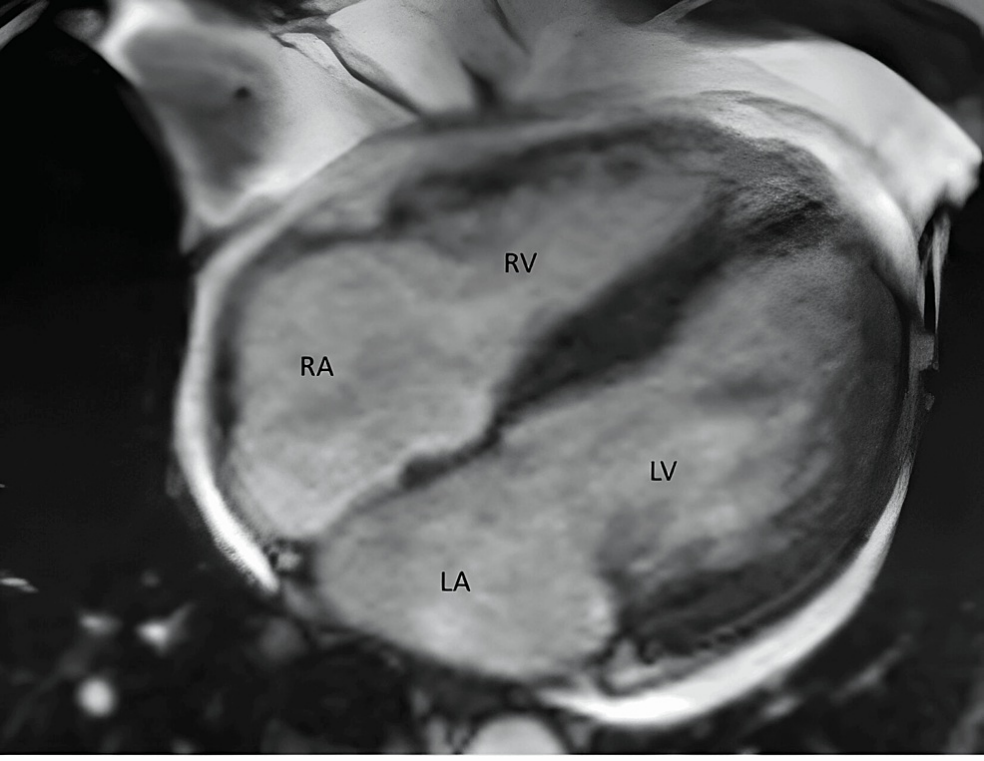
Cardiac magnetic resonance imaging, demonstrating normal systolic function RA, right atrium; LA, left atrium; LV, left ventricle; RV, right ventricle
